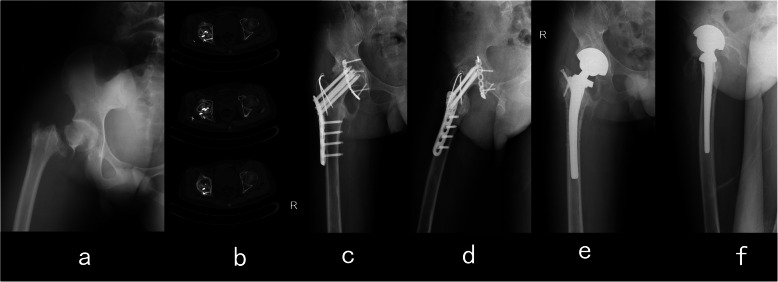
Case 11. A 22-year-old male patient had a traumatic acetabular fracture combined with an ipsilateral intertrochanteric fracture in his right hip. The patient underwent open reduction and internal fixation surgery for both his acetabulum and proximal femur. Then, 2.2 years after surgery, avascular necrosis of the right femoral head was detected, and revision with total hip arthroplasty was performed. a Anterior-posterior view immediately after injury. b Computed tomography image showing avascular necrosis of the right femoral head. c Anterior-posterior view before hip arthroplasty. d Lateral view before hip arthroplasty. e Anterior-posterior view after hip arthroplasty. f Lateral view after hip arthroplasty
